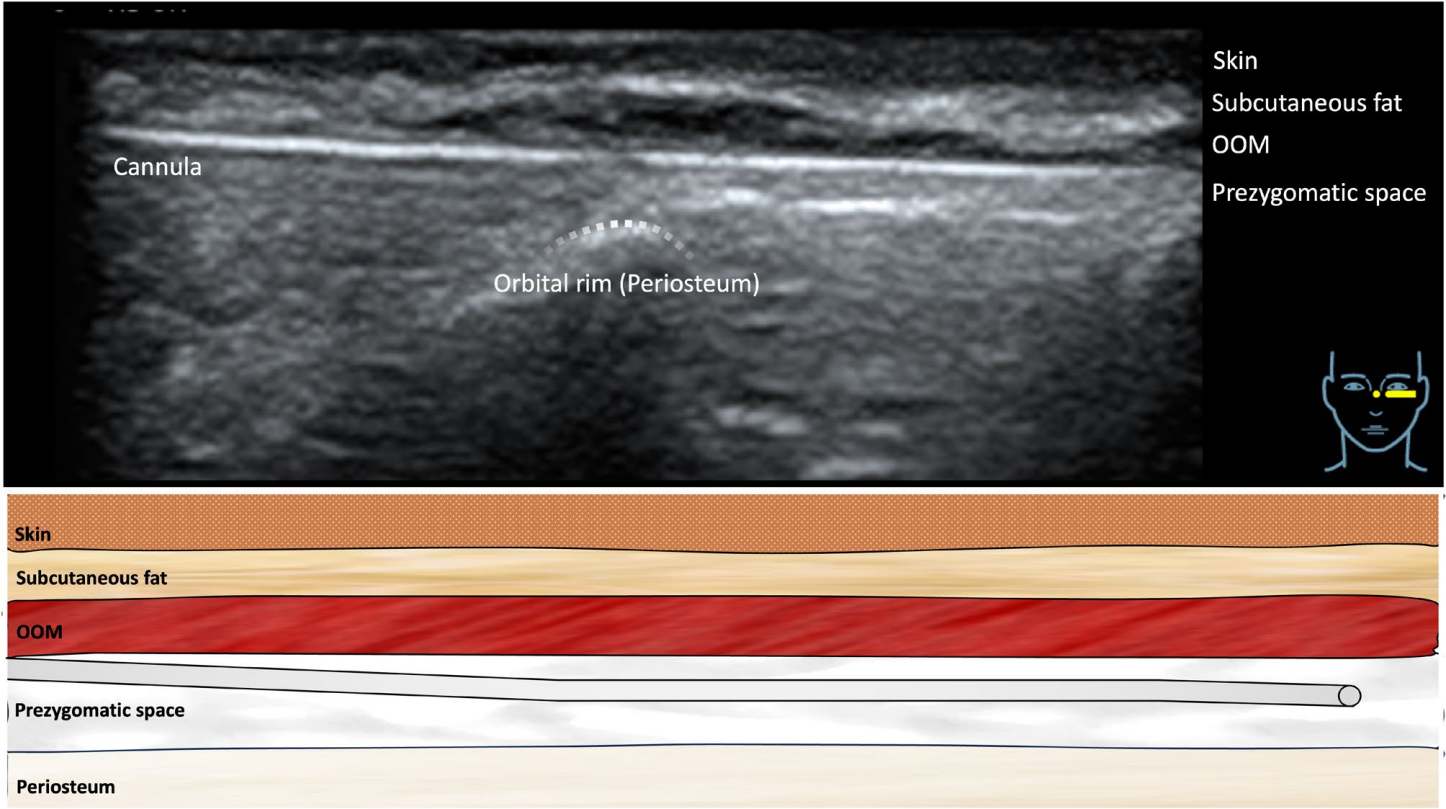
Ultrasound image showing the location of the cannula in the pregzygomatic space just superficial to the orbital rim (= periosteum). The ultrasound transducer was placed in a horizontal fashion.
